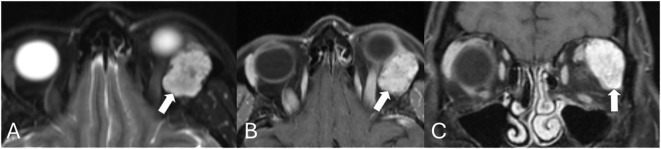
A 45-year-old woman with a left lacrimal pleomorphic adenoma. (A) Axial T2 MRI shows a multilobulated iso- to hyperintense appearance of the lacrimal mass (arrow). (B, C) Axial and coronal T1 post-contrast MRI shows an oval-shaped mass with heterogeneous enhancement that extends posteriorly in the orbit without bony involvement (arrows).
